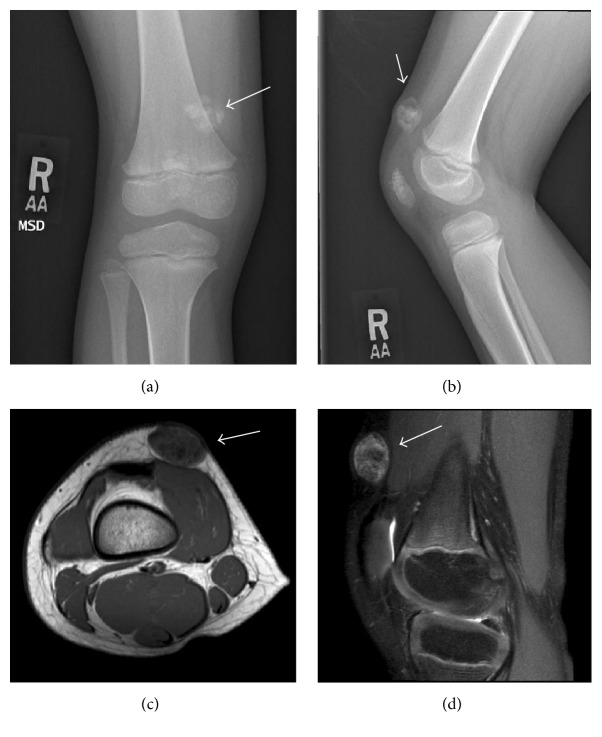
AP (a) and lateral (b) radiographic imaging of distal femur demonstrates aberrant calcification of the suprapatellar region within the soft tissue. Transverse (c) and lateral magnetic resonance imaging (d) reveals that the calcified mass is confined to the subcutaneous tissue with no connections to the underlying bone.
